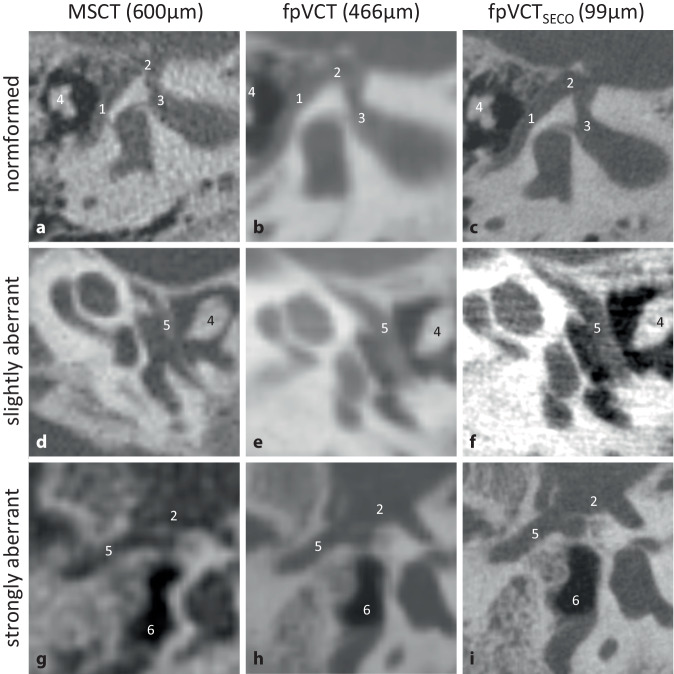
Visualization of the course of the facial nerve. A representative image with a MSCT (600 µm slice thickness), b fpVCT (466 µm slice thickness), and c fpVCT_SECO_ (99 µm slice thickness). Respective images d–f demonstrating a slightly aberrant course of the facial nerve through the tympanic cavity and g–i a strongly anomalous course of the facial nerve. *1* Canalis nervi facialis, *2* ganglion geniculatum, *3* meatus acusticus internus, *4* malleus incus complex, *5* dysmorphic course of the facial nerve, *6* dysmorphic reduced tympanic cavity. *MSCT* multislice computed tomography, *fpVCT* flat-panel volume computed tomography, *fpVCT*_*SECO*_ secondary reconstructions of fpVCT
